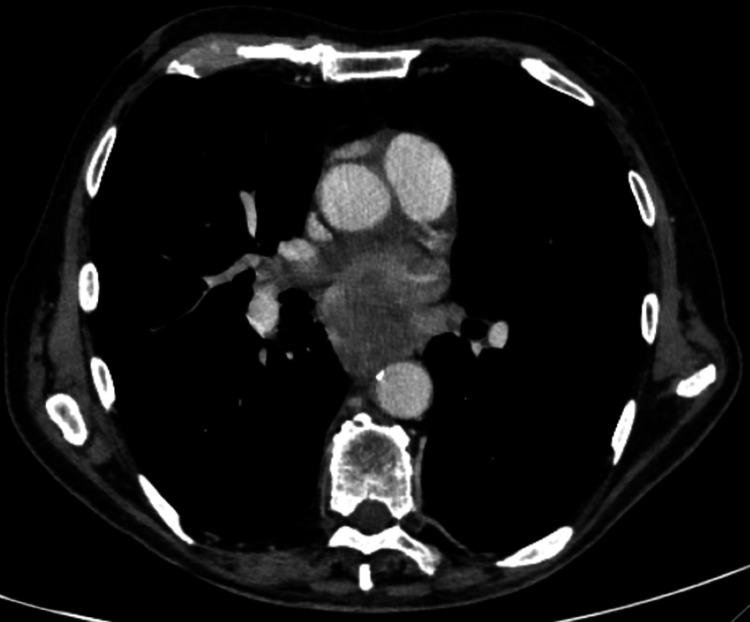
CTTAP revealing an ill-defined oesophageal mass encasing the left main bronchus CTTAP: CT thorax, abdomen, and pelvis
